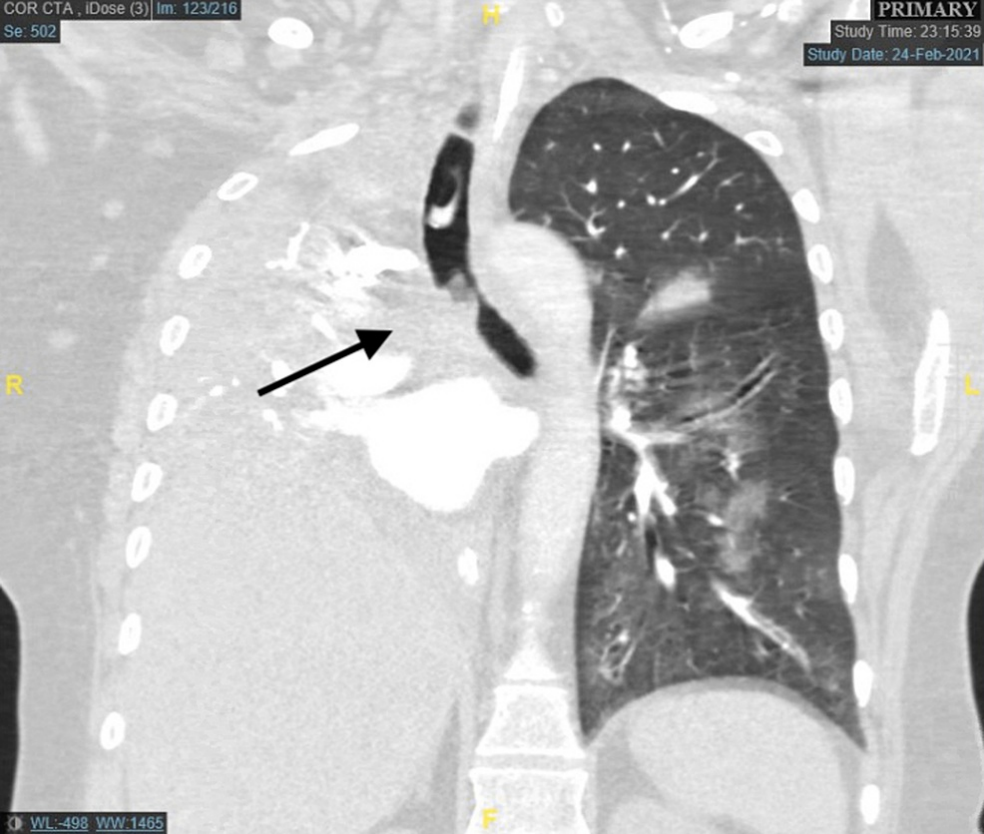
Chest computed tomography showing a right hilar mass invading the right main stem and lower trachea with complete right lung collapse.
